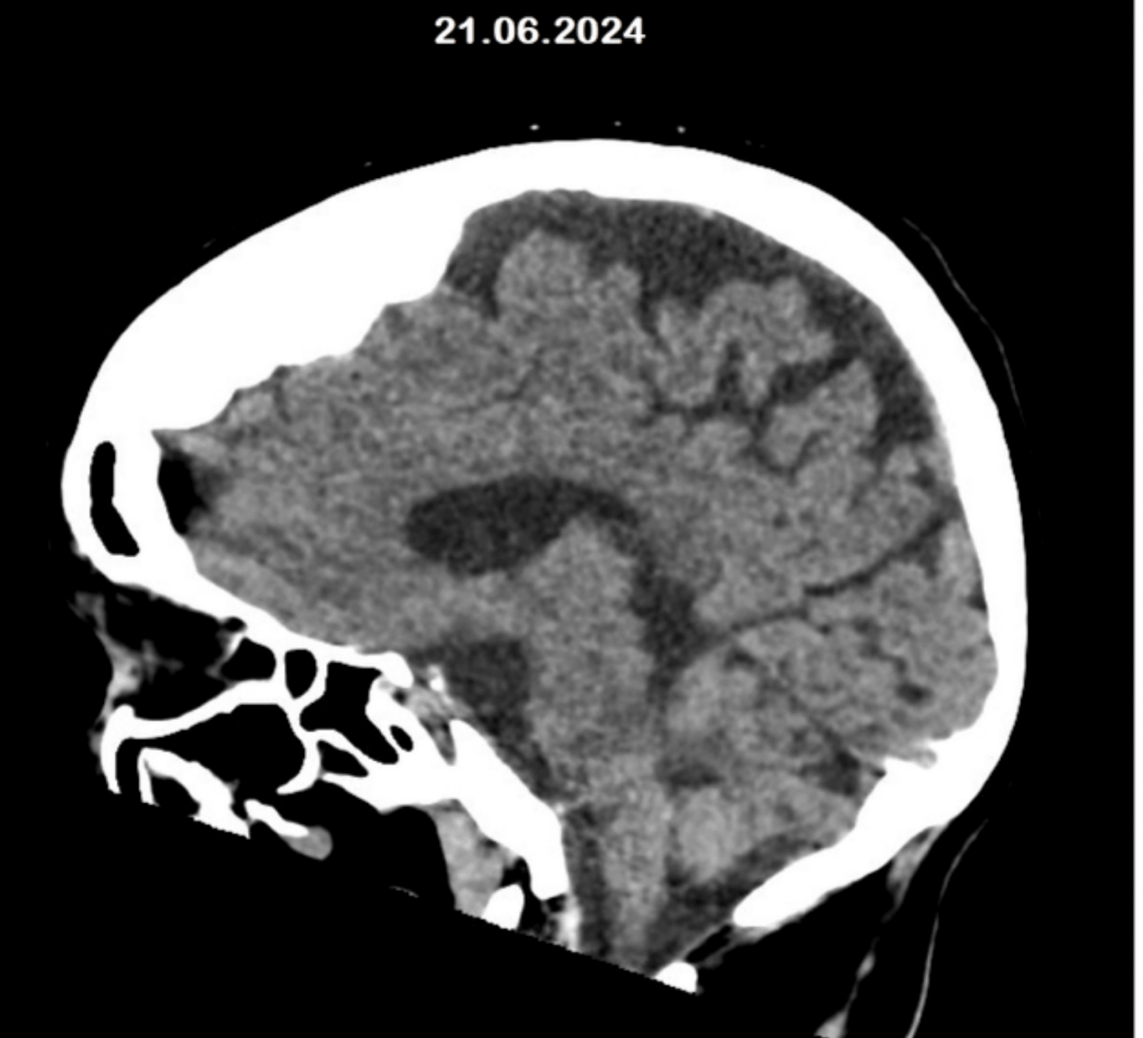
Non-contrast CT scan of the head. A non-contrast CT scan of the head was done at the time of admission as part of the frailty and confusion screen.
